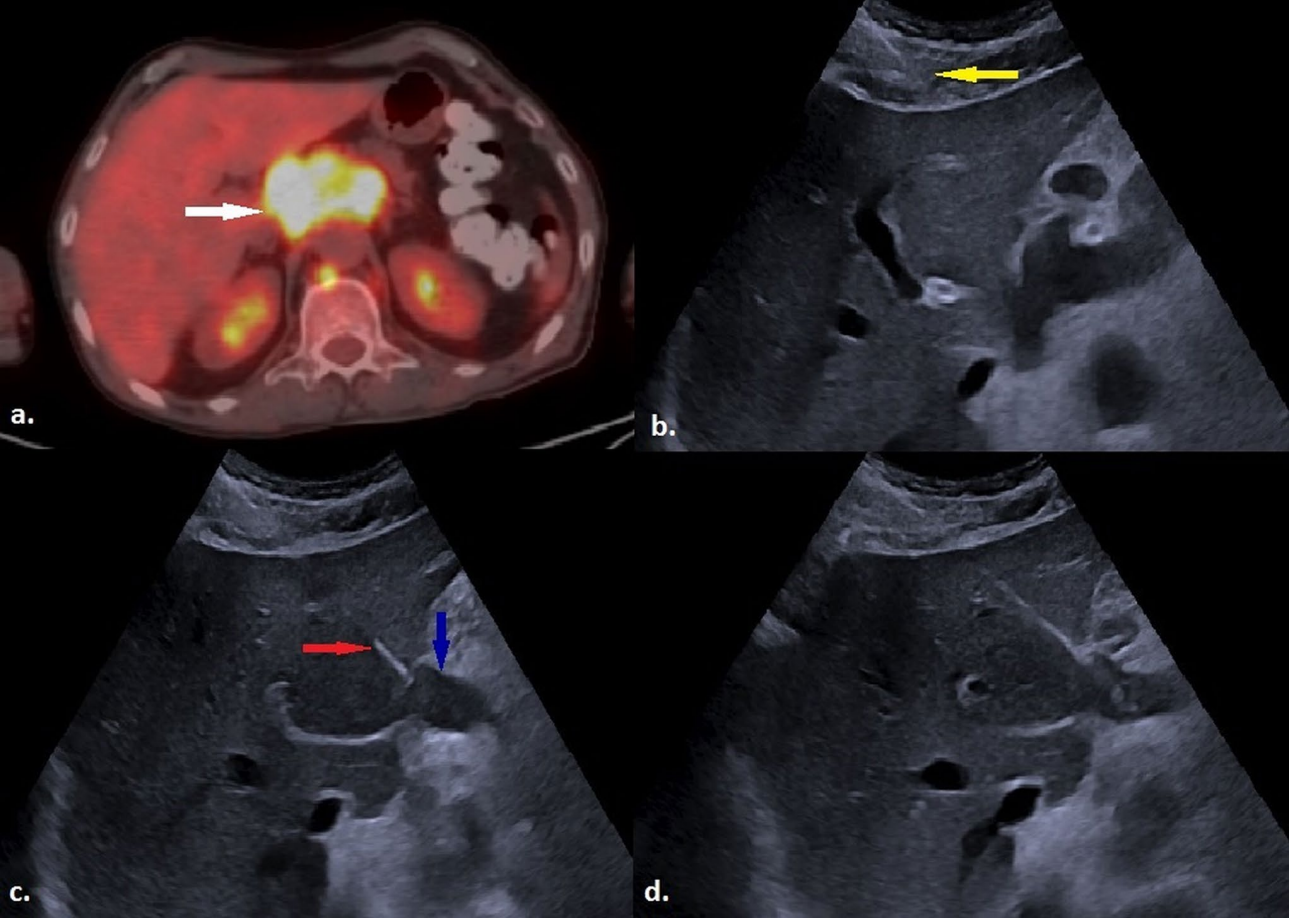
a) An axial PET/CT image shows conglomerate lymphadenopathy with high SUV uptake in the porta hepatis region (white arrow). b) Prior to the biopsy, local anesthesia is administered with 2% prilocaine hydrochloride using a 21-gauge needle to the subcutaneous tissue and around the anterior liver capsule (yellow arrow). c) A hypoechoic lymph node in the portal hilum (blue arrow) is accessed via CTHa using a 17-gauge introducer (red arrow). d) Ultrasound image obtained immediately after the biopsy procedure. Pathological examination of the lesion confirmed Hodgkin lymphoma. (PET/CT: Positron Emission Tomography / Computed Tomography; CTHa: Complete Transhepatic Approach; SUV: Standardized Uptake Value)
